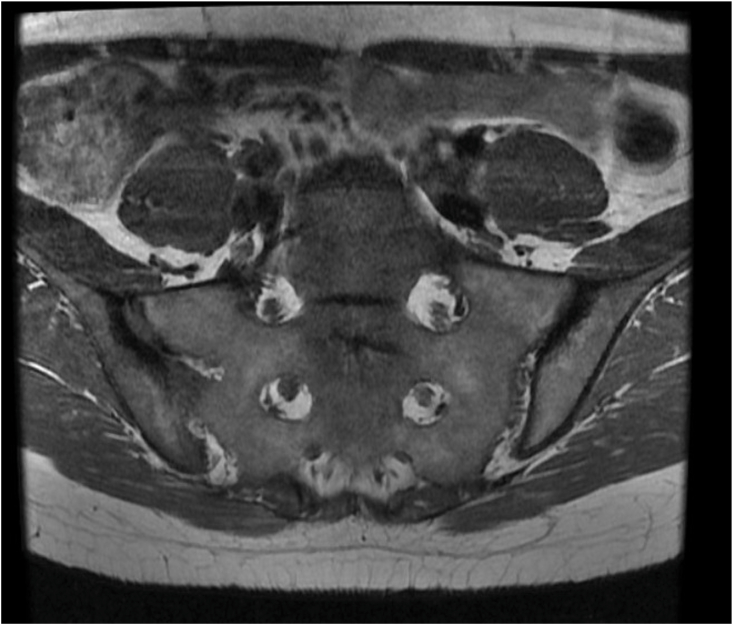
Pelvic MRI findings: T1-weighted coronal image showing bilateral subchondral sclerosis of the sacroiliac joints.
